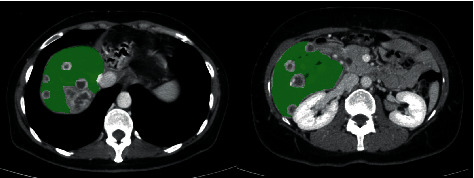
Exemplary contrast-enhanced axial CT slices in the portal venous phase depicting the volumetric measurement of tumor-free liver tissue for one patient using Aquarius iNtuition® software.
